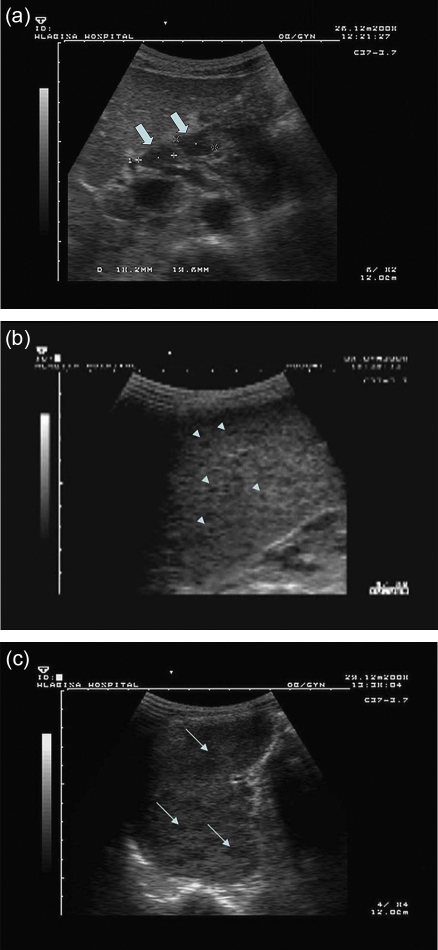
Sonographic image of (a) enlarged lymph nodes in the periportal area; (b) focal lesions in the spleen of approximately 3 mm in diameter; (c) focal lesions in the spleen of approximately 10 mm in diameter.
